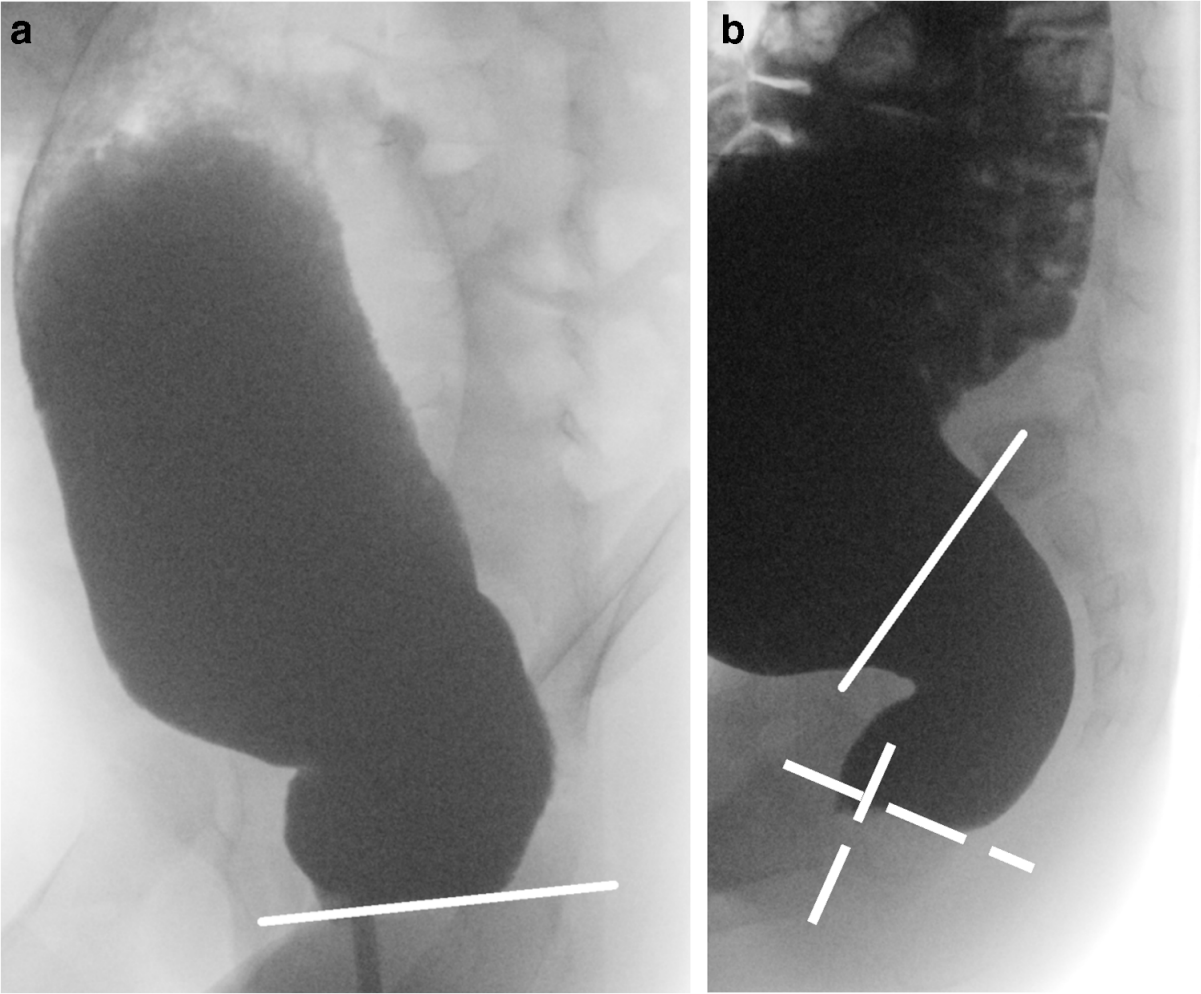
Lateral radiographs of the rectum obtained during a defecography performed to determine the transition zone in a 3.5-year-old girl with Hirschsprung disease. a There is no rectoanal inhibitory reflex detectable since there is no bulge below the tangent (*line*) on the caudal rectal contour. b During active defecation, there is no opening of the anal channel and no reduction in the anorectal angle (*broken*
